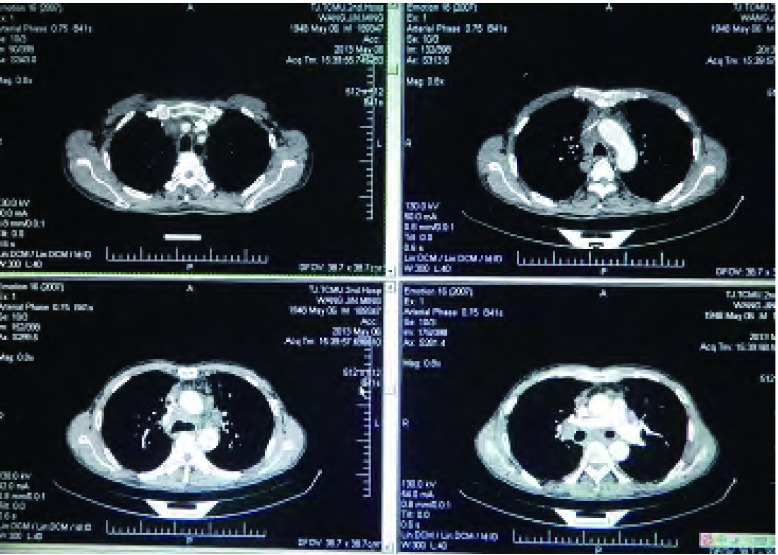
胸部CT示：纵膈密度增高，其内组织分界欠清并伴多发淋巴结增大 The chest CT showed: mediastinal density increased, obscure boundary with multiple enlarged lymph nodes. CT: computed tomography.
